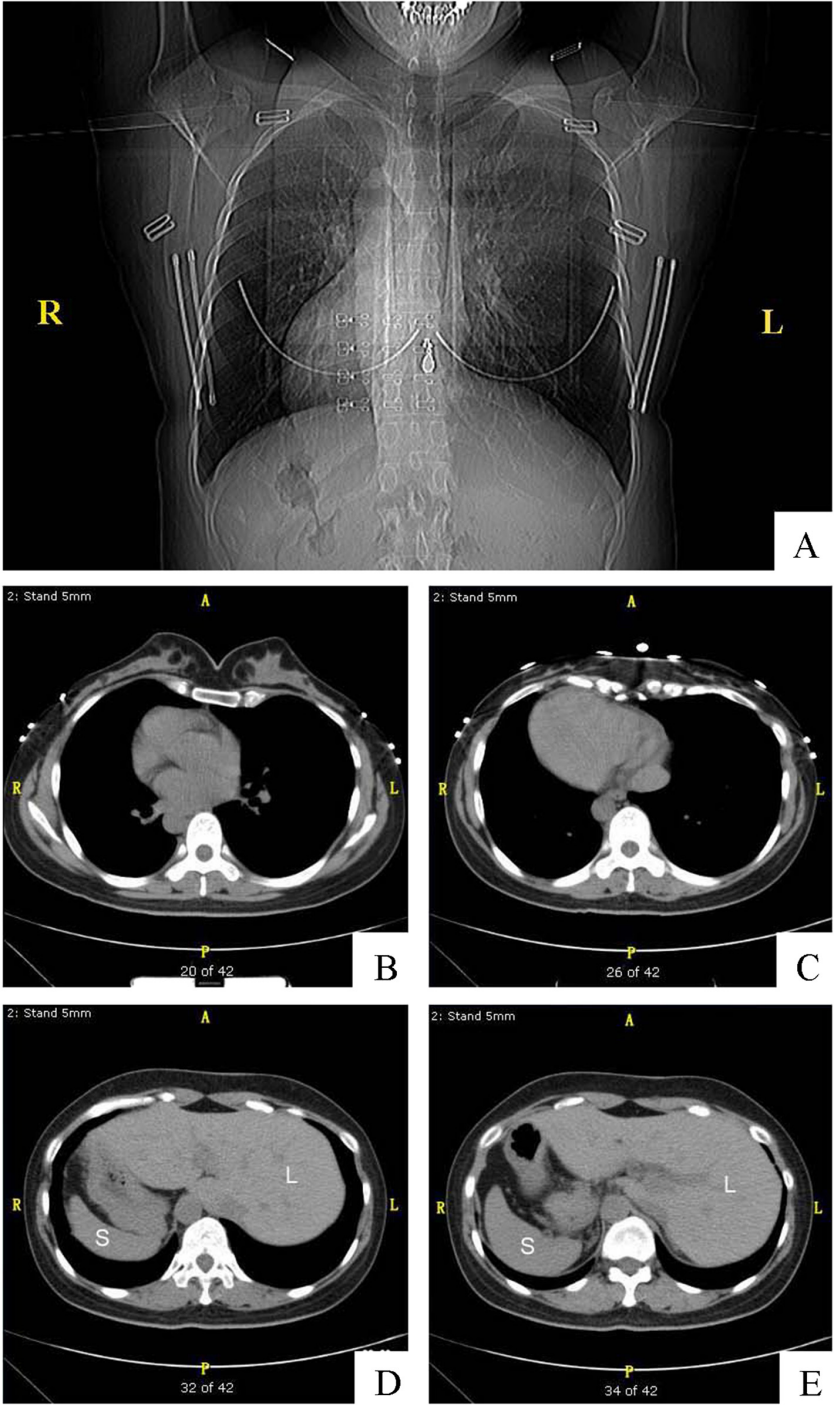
The computed tomography image of the chest demonstrated a normal-sized heart with dextrocardia, right-sided gastric air bubble, aortic knob and descending aorta. The left hemidiaphragm was higher than the right one. This picture was compatible with situs inversus totalis. a Thoracic computed tomography scan shows the dextrocardia (b and c). The computed tomography image of the abdomen. CT scan shows the liver on the left side and spleen on the right, which confirms the presence of situs inversus totalis. Note the situs inversus anatomy of the abdominal organs. L: Liver S: Spleen (d and e)
